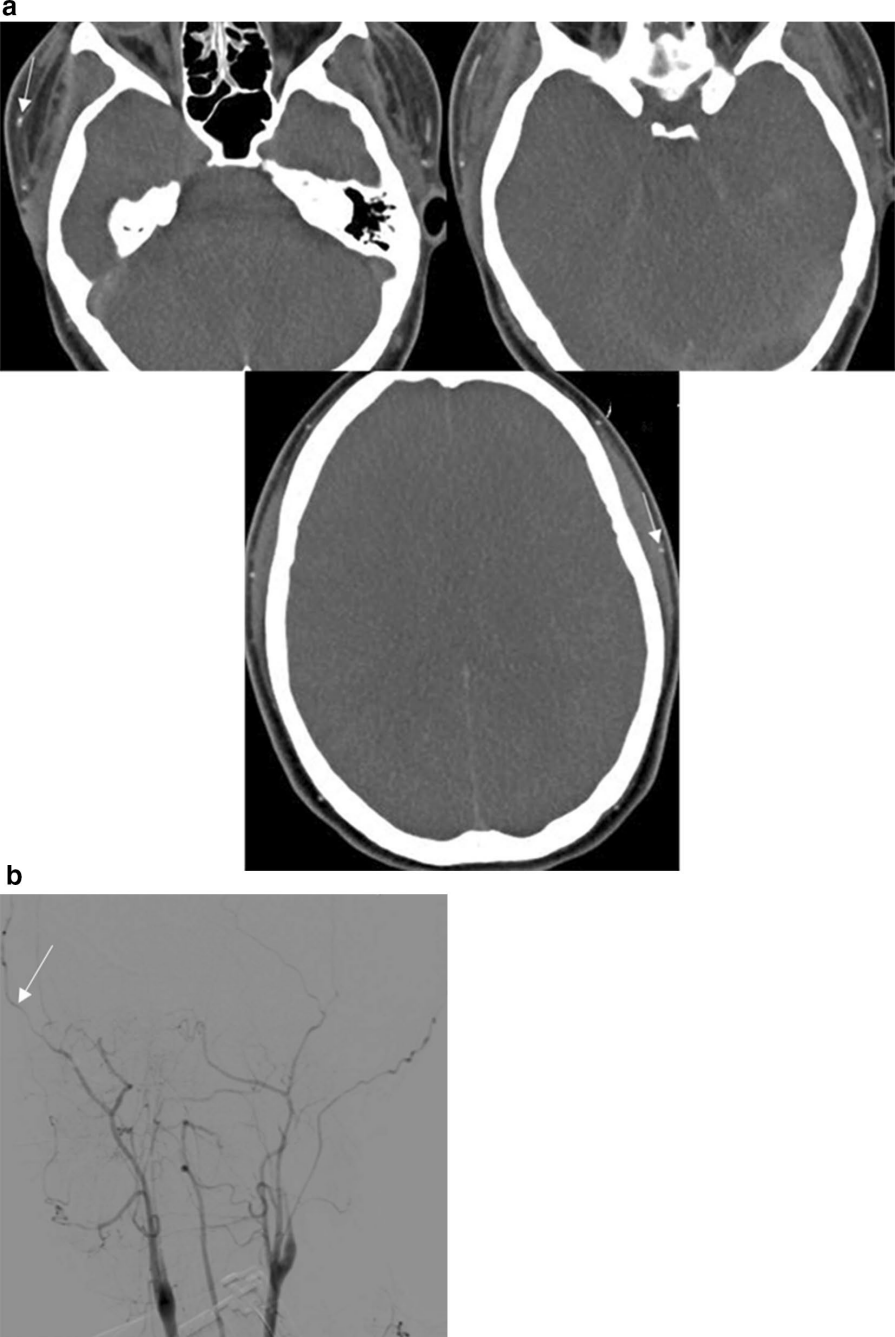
(a) Axial CTA (60 s phase) at different levels, shows no intracranial arterial flow or venous flow. Extracranial arterial feeders are visible (white arrows). (b) Digital Subtraction Angiography in arterial phase (AP view), shows no intracranial flow, while extracranial arteries are patent (white arrow).
